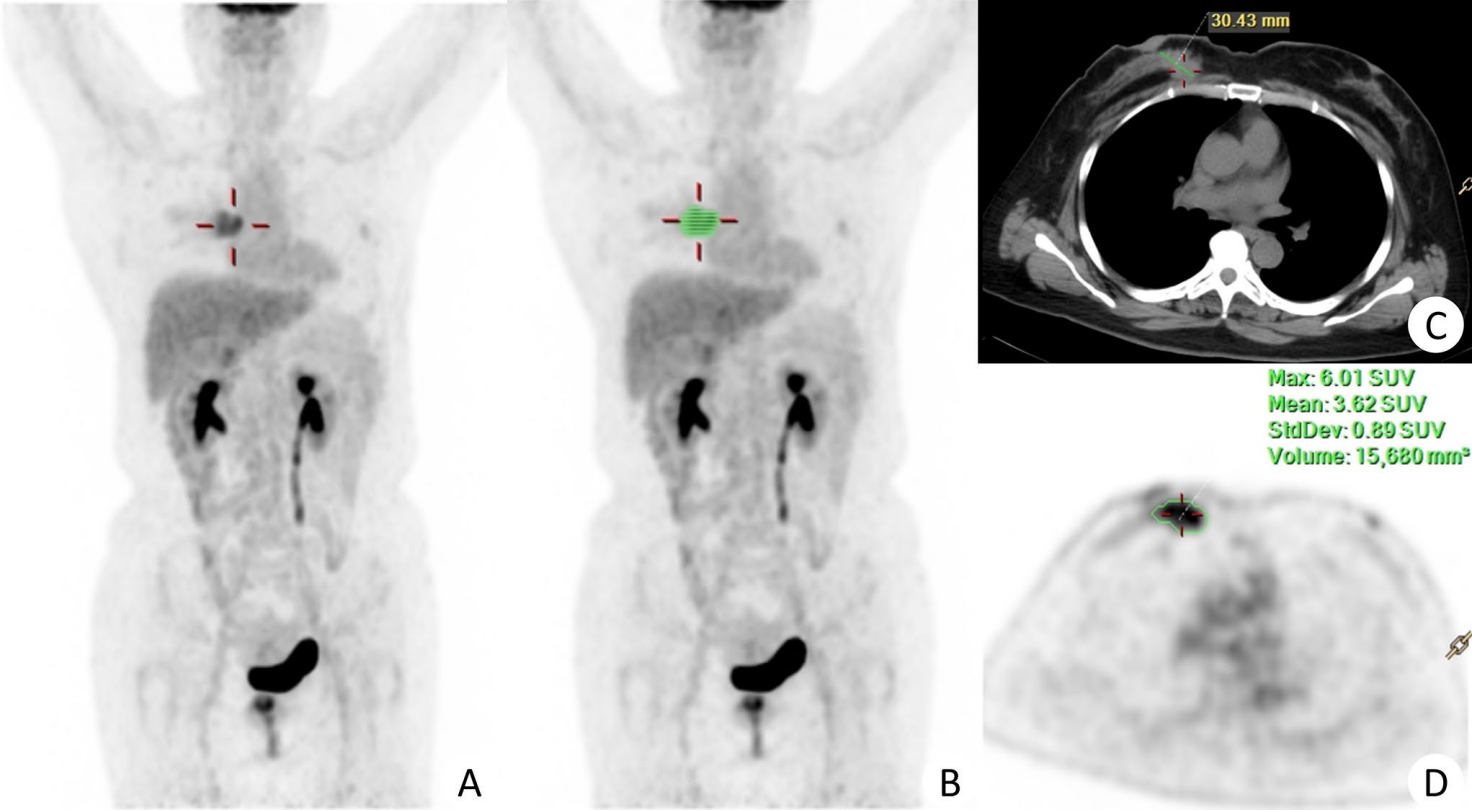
The delineation of the VOI of BC. A female, 56 years old, with breast cancer on the right side, underwent an ^18^F-FDG PET/CT scan. (A) (MIP) showed a high uptake of ^18^F-FDG of the cancer. The VOI was segmented with a 40% SUVmax on the EBW workstation (B). The longest diameter of the cancer was measured on the maximal horizontal position and was 30.43 mm (C). The SUVmax, SUVmean, SD, and MTV were 6.01, 3.62, 0.89, and 15.680mm^3^ (D)
